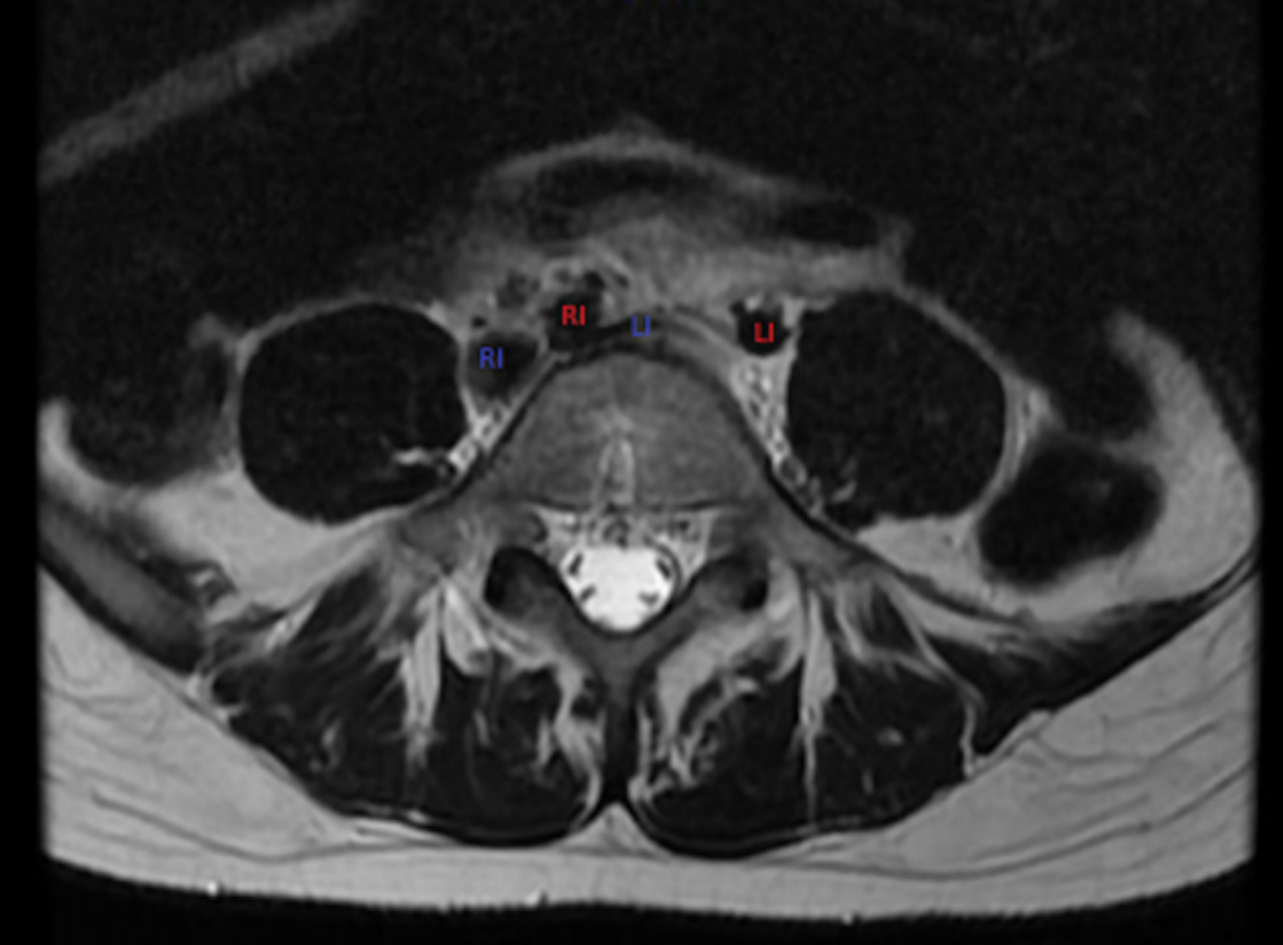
MRI of the lumbar spine again noting flattening of the left common iliac vein (blue LI) by the right iliac artery (red RI). Right common iliac vein (blue RI) and left iliac artery (red LI) also noted.
